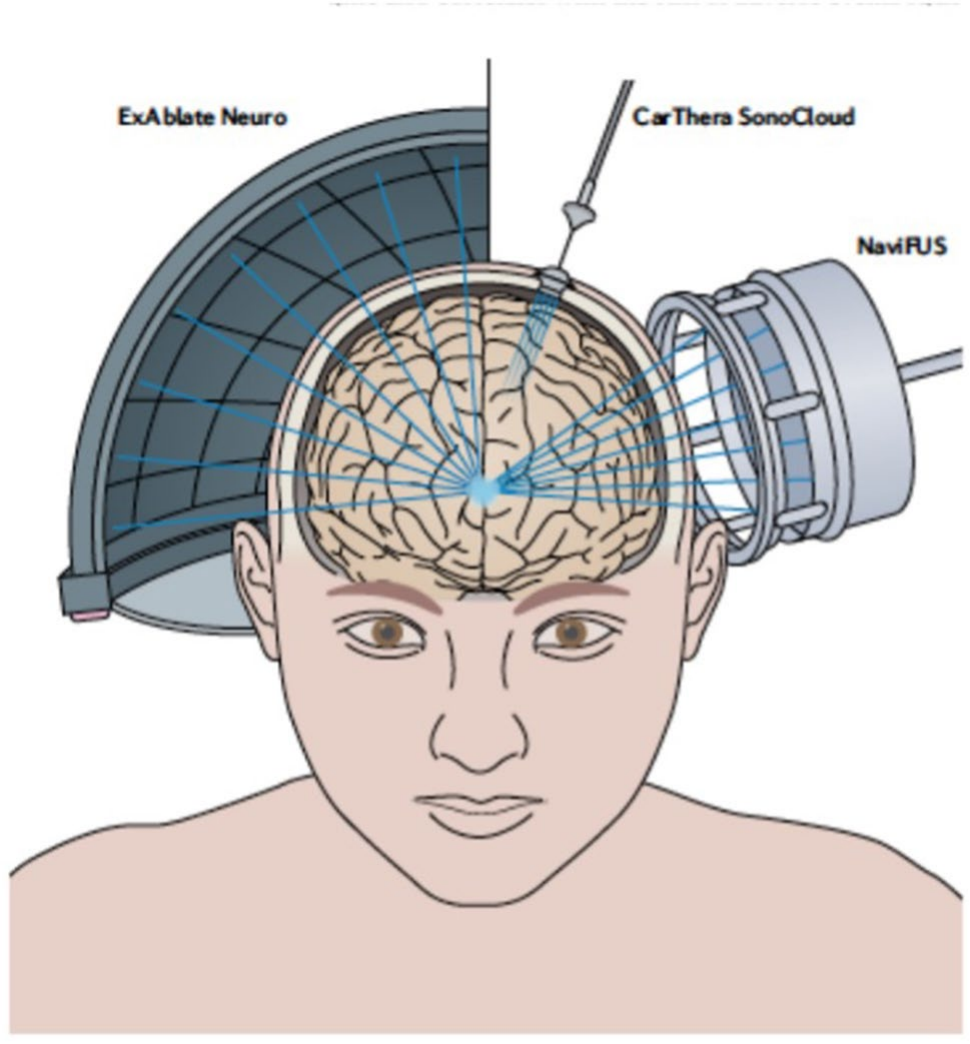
Focused Ultrasound Devices for GBM Treatment. The ExAblate Neuro device uses MRI images during the procedure to guide the focused ultrasound beam. The NaviFUS system is guided by neuronavigation with a previously performed MRI scan to guide the beam to the target region; it can be performed in an office setting. The CarThera SonoCloud device is implanted in the skull overlying the target region and does not use imaging guidance. Image used with Copyright Clearance Center permission from Meng Y, Hynynen K, Lipsman N, *Nat Rev Neurol*. 2021;17(1):7–22 [46]
